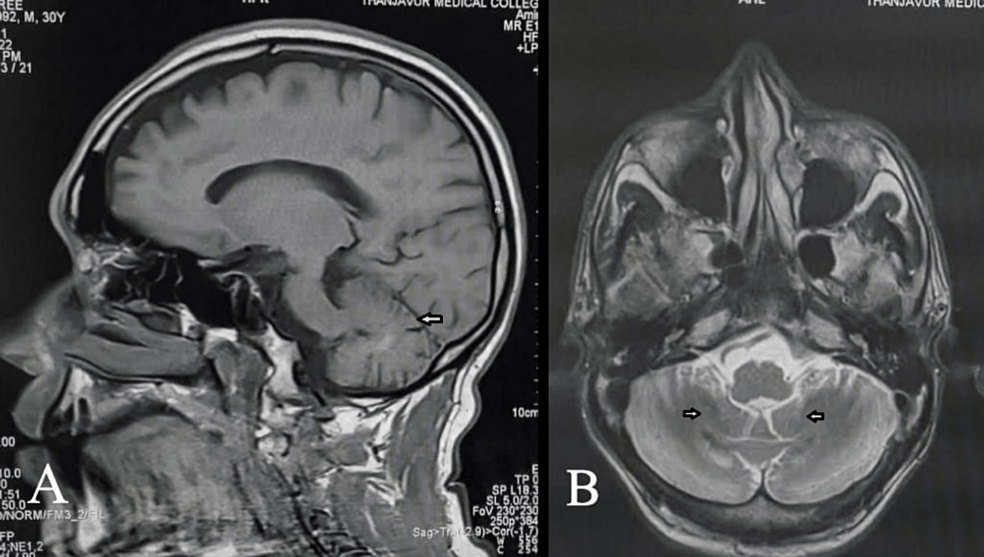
MRI brain finding of case 1 MRI brain revealed (A) T2 sagittal section showing cerebellar atrophy (arrow) and (B) T2 axial section showing bilateral symmetrical hypointensities involving dentate nuclei suggesting calcification (arrows)
